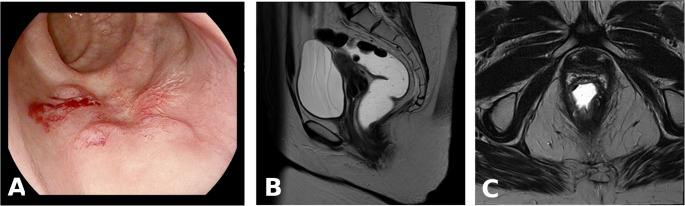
Findings of (A) endoscopic (irregular mucosal surface of the former tumor bed) vs. MRI image (radiological complete response (B) T2 sagittal, (C) T2 axial)
